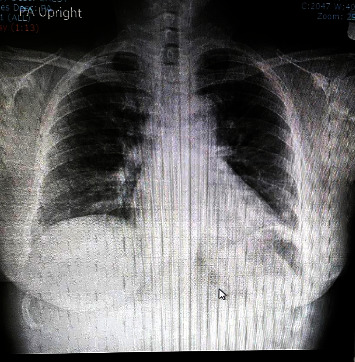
Chest radiography on admission.
